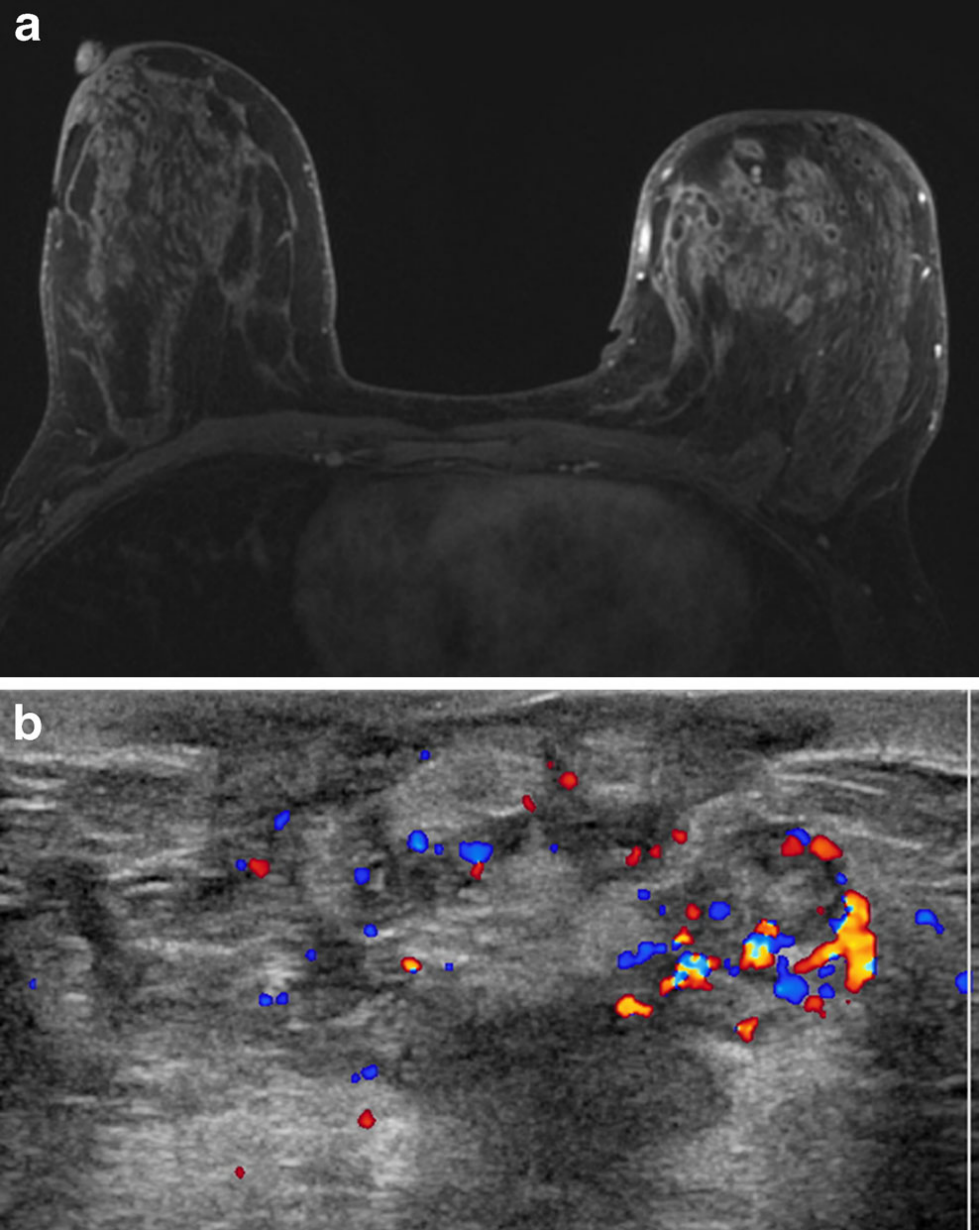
A 39-year-old woman treated with multiple prior incision and drainages of the lateral lower left breast, presenting with new pain and palpable thickening left inner upper breast. a Post-contrast, vibrant, T1-weighted, fat-saturated axial MRI image showing extensive rapid enhancement of the left inner breast with central areas of non-enhancement consistent with microabscess formation. b US of the same area shows multiple serpiginous hypoechoic areas with increased vascularity noted on color Doppler evaluation
